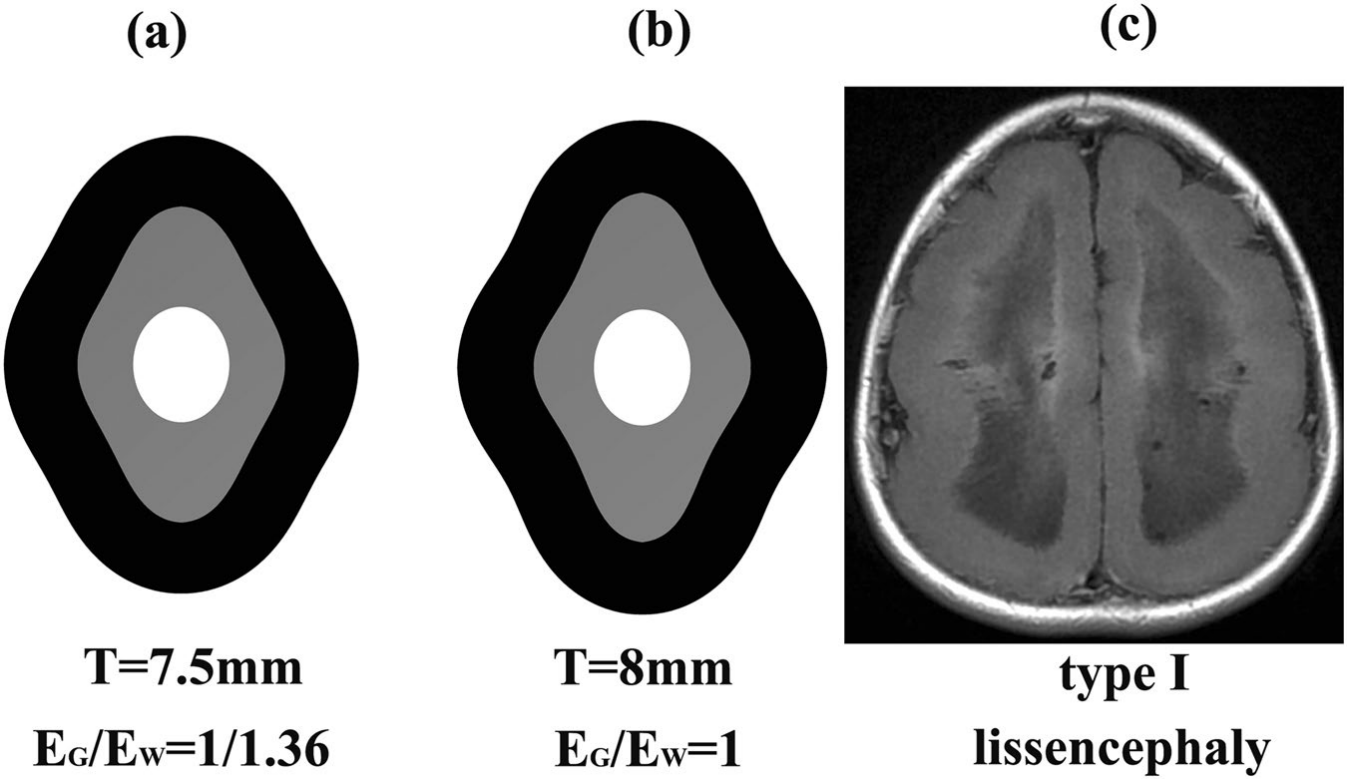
The lissencephaly. (a) The cortical folding at stiffness ratio E_G_/E_W_ = 1/1.36 and initial cortical thickness T = 7.5 mm. (b) The cortical folding at stiffness ratio E_G_/E_W_ = 1 and initial cortical thickness T = 8 mm. (c) The MRI of type І lissencephaly. Case courtesy of Dr. Amro Omar, Radiopaedia.org, rlD: 32628. When the thickness was 7.5 mm (E_G_/E_W_ = 1/1.36) and 8 mm (E_G_/E_W_ = 1), the cortical folding became type І lissencephaly. The stiffer the white matter, the more easily the lissencephaly appears.
